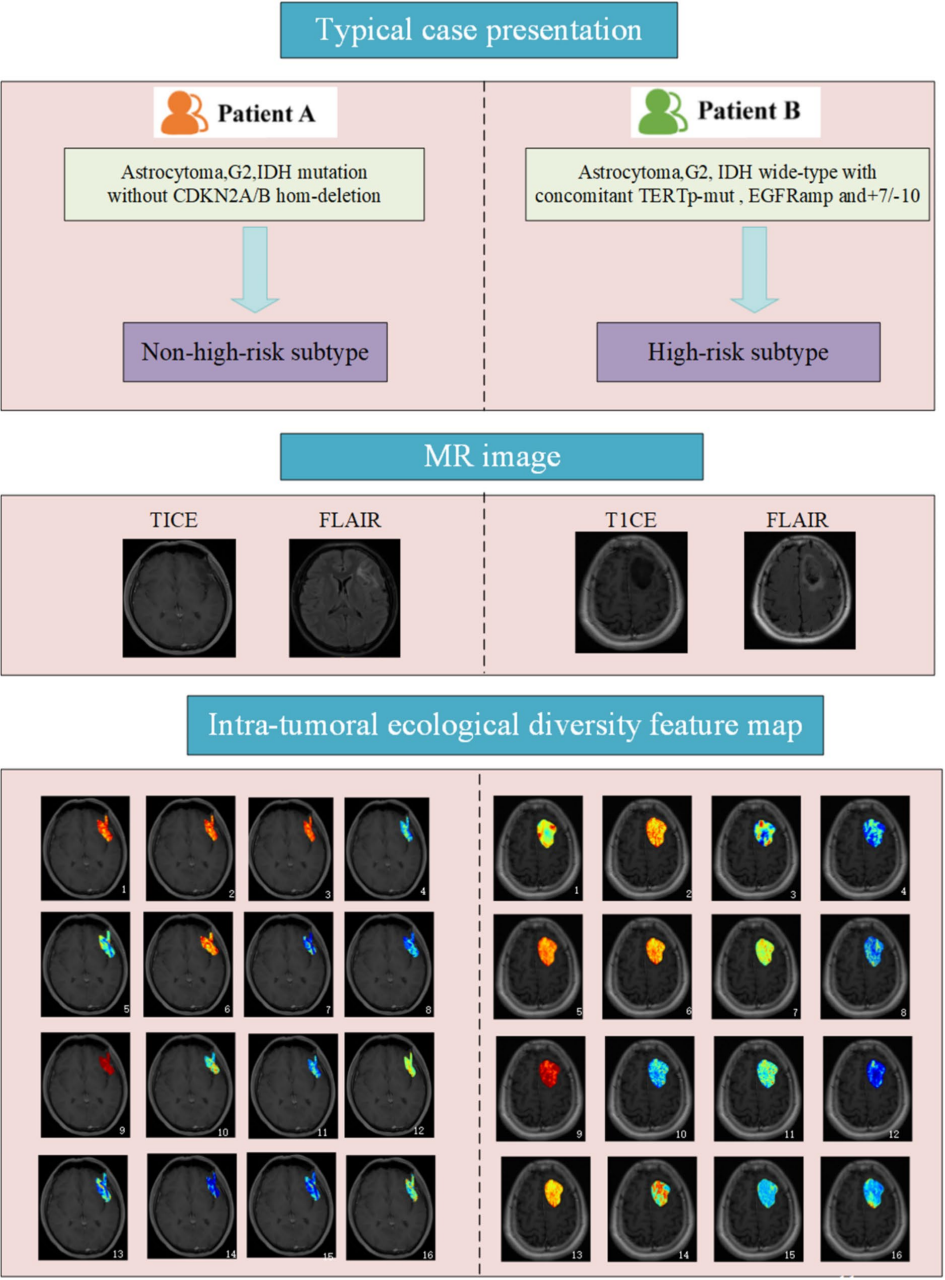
This figure depicts the MRI images and habitat feature maps of two patients with diffuse astrocytoma (WHO grade 2) from different molecular subtypes. Preoperative conventional MRI of patients (A) and (B) shows similar radiological features, with no enhancement on CE-T1WI images and slightly heterogeneous high signal on T2-FLAIR images. However, the habitat feature maps of these two patients exhibit significant differences
